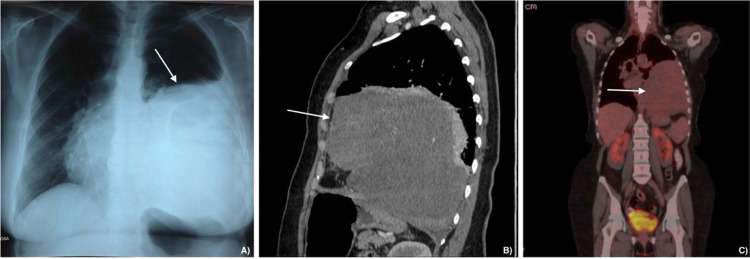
Preoperative imaging of the initial tumor. (A) Chest radiograph (posteroanterior view), (B) computed tomography (CT) sagittal reconstruction, and (C) positron emission tomography-computed tomography (PET-CT) coronal reconstruction of the first tumor, obtained ten years before the current presentation. Imaging demonstrates a large, well-circumscribed, homogeneous opacity occupying the left mid-to-lower hemithorax, with convex lateral margins and effacement of the left cardiac border and hemidiaphragm (silhouette sign), consistent with a pleural-based mass.
